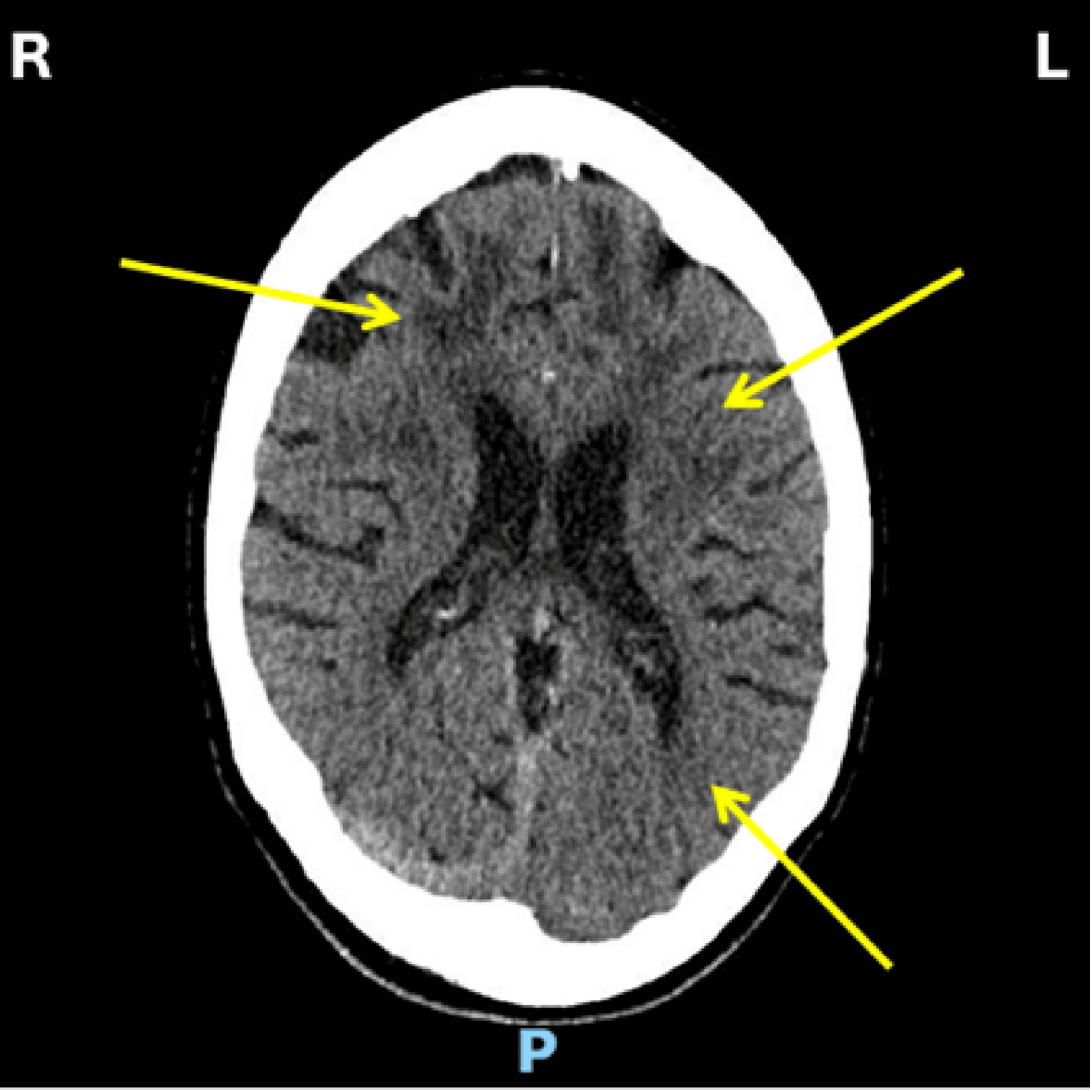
Axial non-contrast CT head demonstrating small vessel disease (yellow arrows) CT: computed tomography CT head showing confluent white matter low attenuation periventricular areas in keeping with small vessel disease ischaemic change
